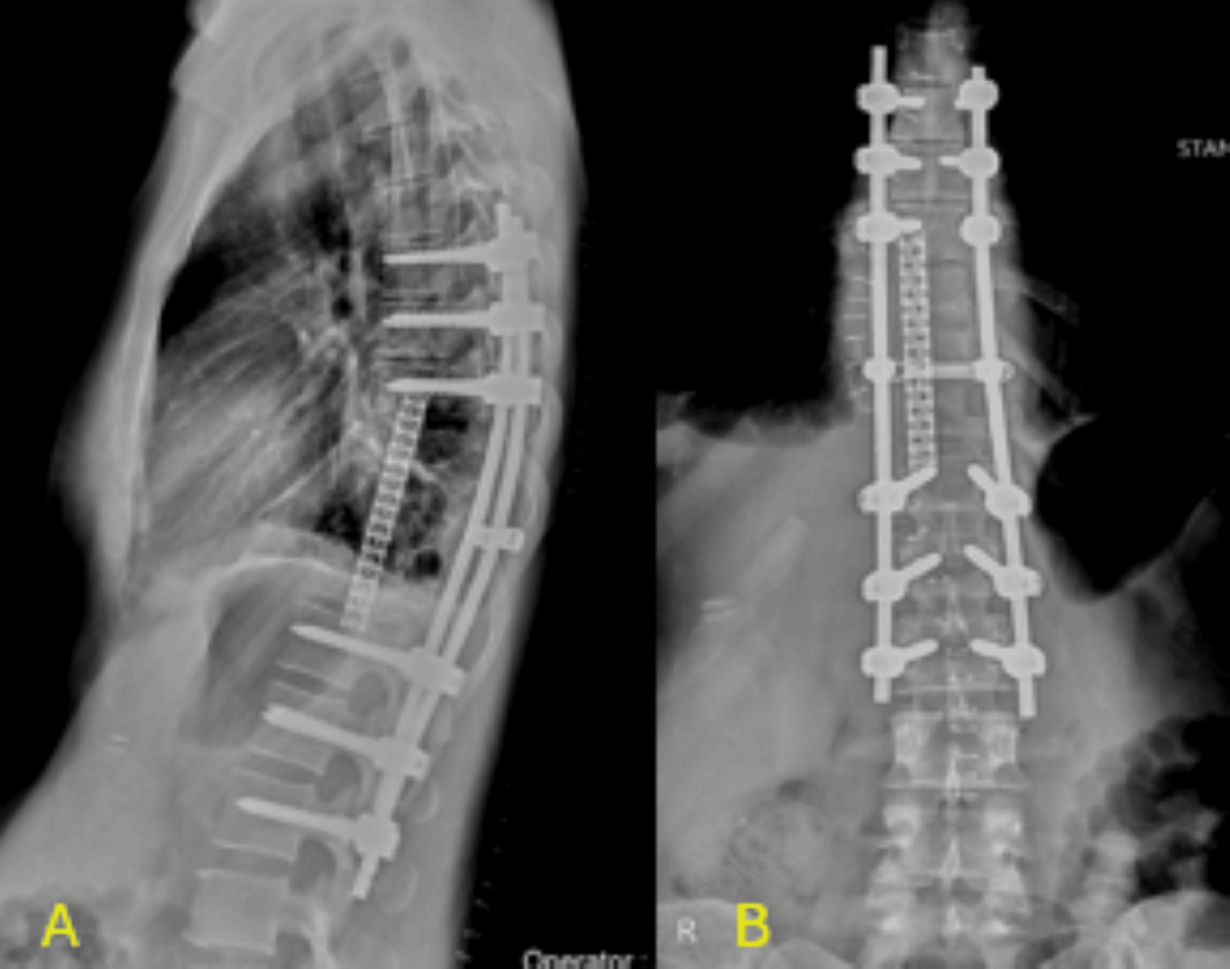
Post-operative X-rays Immediate post-operative x-rays showing the mesh cage anteriorly and posterior instrumentation 4A: Sagittal X-rays showing the mesh cage anteriorly and posterior instrumentation 4B: Coronal X-rays showing the mesh cage and spinal instrumentation
